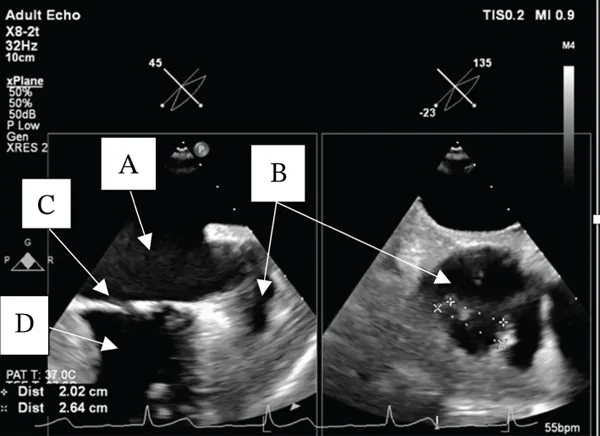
Echocardiographic images of left atrial appendage in 45° and 135° short axis view. The 45° short axis view shows (A) left atrium, (B) left atrial appendage, (C) mitral valve, and (D) left ventricle. The 135° short axis view shows (B) left atrial appendage.
